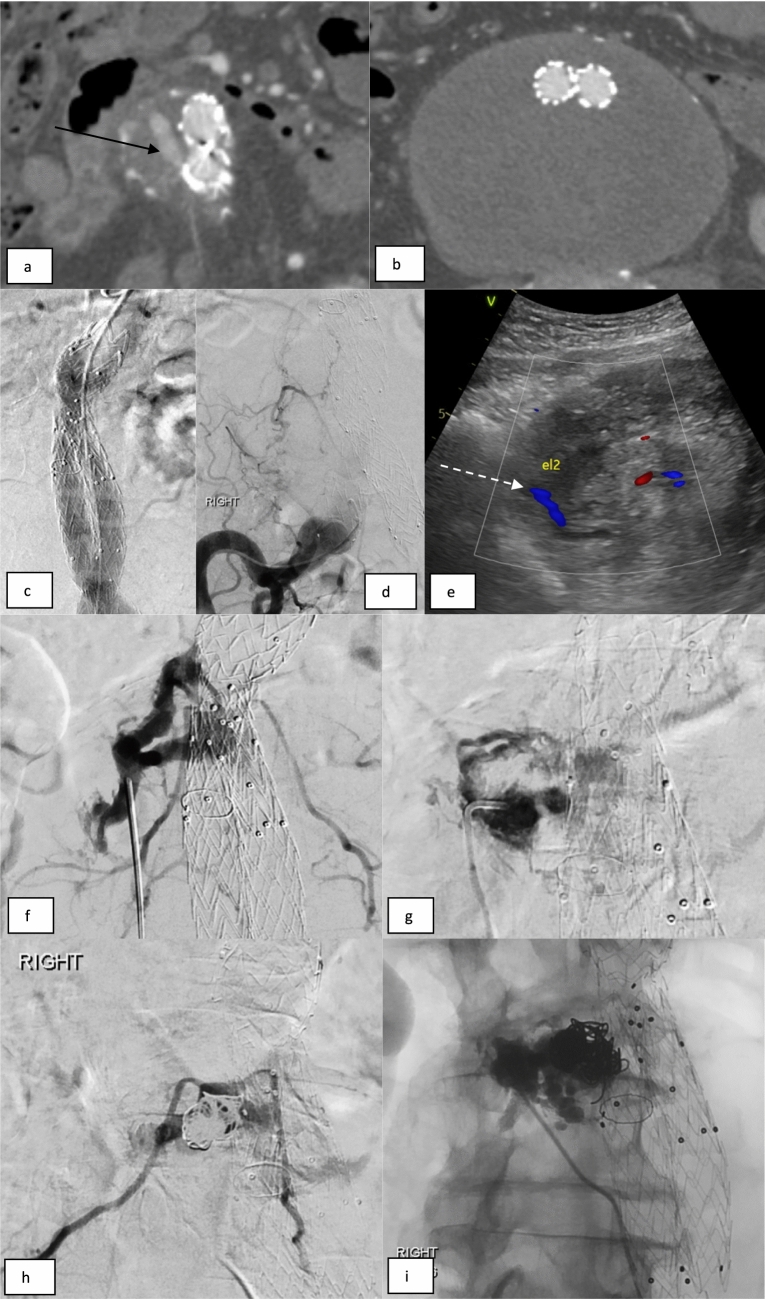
Direct sac puncture with embolisation of bilateral lumbar T2ELs. (a, b) CTA imaging demonstrates an endoleak of unclear source (arrow). (c, d) Catheter angiograms from the aorta and right common iliac artery do not show a clear endoleak. (e) A duplex ultrasound demonstrates a T2EL endoleak in the aneurysm sac (dashed white arrow) and confirms a safe percutaneous transabdominal window for access. (f) Access was obtained under direct US guidance with an 18G trocar needle. Angiogram from the aneurysm nidus demonstrates contrast opacification of the aneurysm sac and bilateral lumbar ‘outflow’ branches. (g) An angiogram from a 4Fr sheath and catheter sited in the aneurysm sac. (h) Several pushable coils were placed within the aneurysm sac prior to liquid embolisation. (i) 4mls of NBCA injected via the catheter spreads into the sac and out into the lumbar vessels bilaterally
